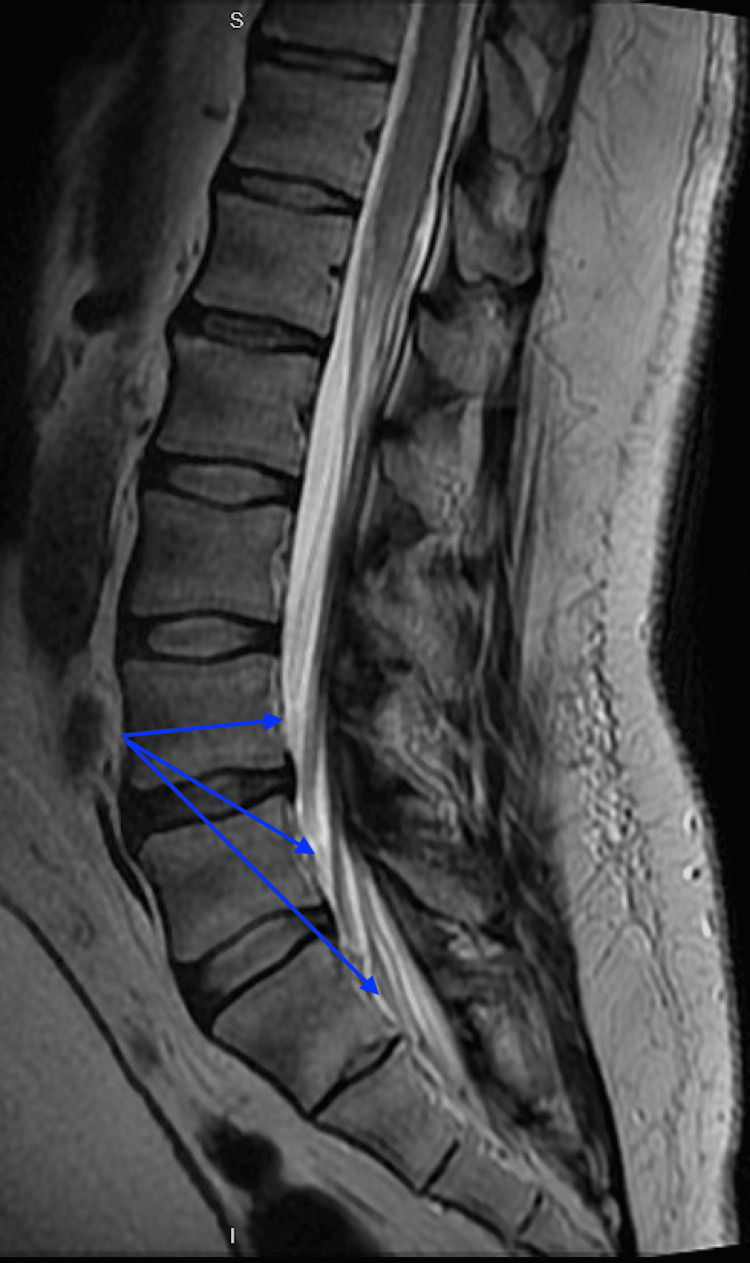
MRI of the lumbar spine showing nodular contiguous ventral and dorsal leptomeningeal enhancement (blue arrows)
